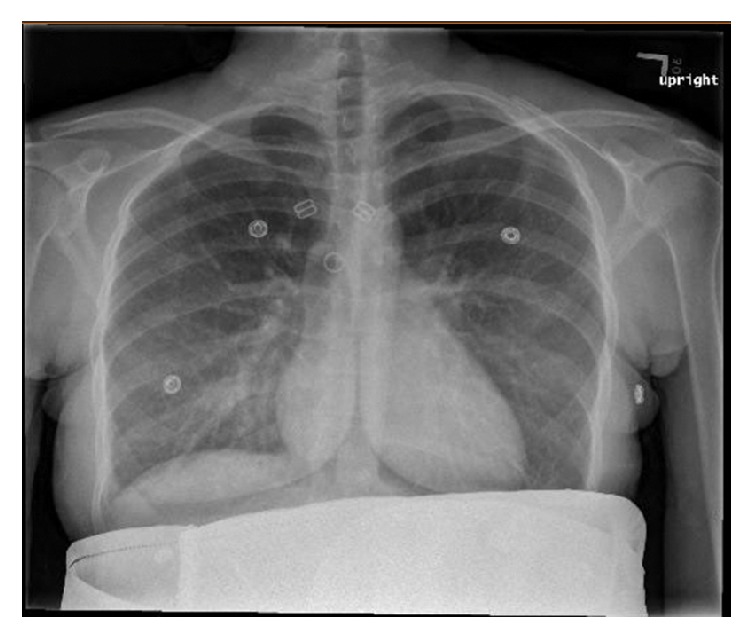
Chest radiograph on the day of admission noted indistinct increased markings and a patchy opacity in the right lower lung.
